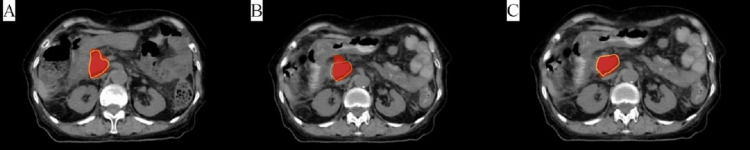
Dose distribution for 45Gy, showing target volume coverage of different plans. (A) Dose distribution of the initial plan. (B) Recalculation of the initial plan on FBCT images during online ART. (C) Adaptive plan FBCT: fan-beam computed tomography; ART: adaptive radiotherapy
